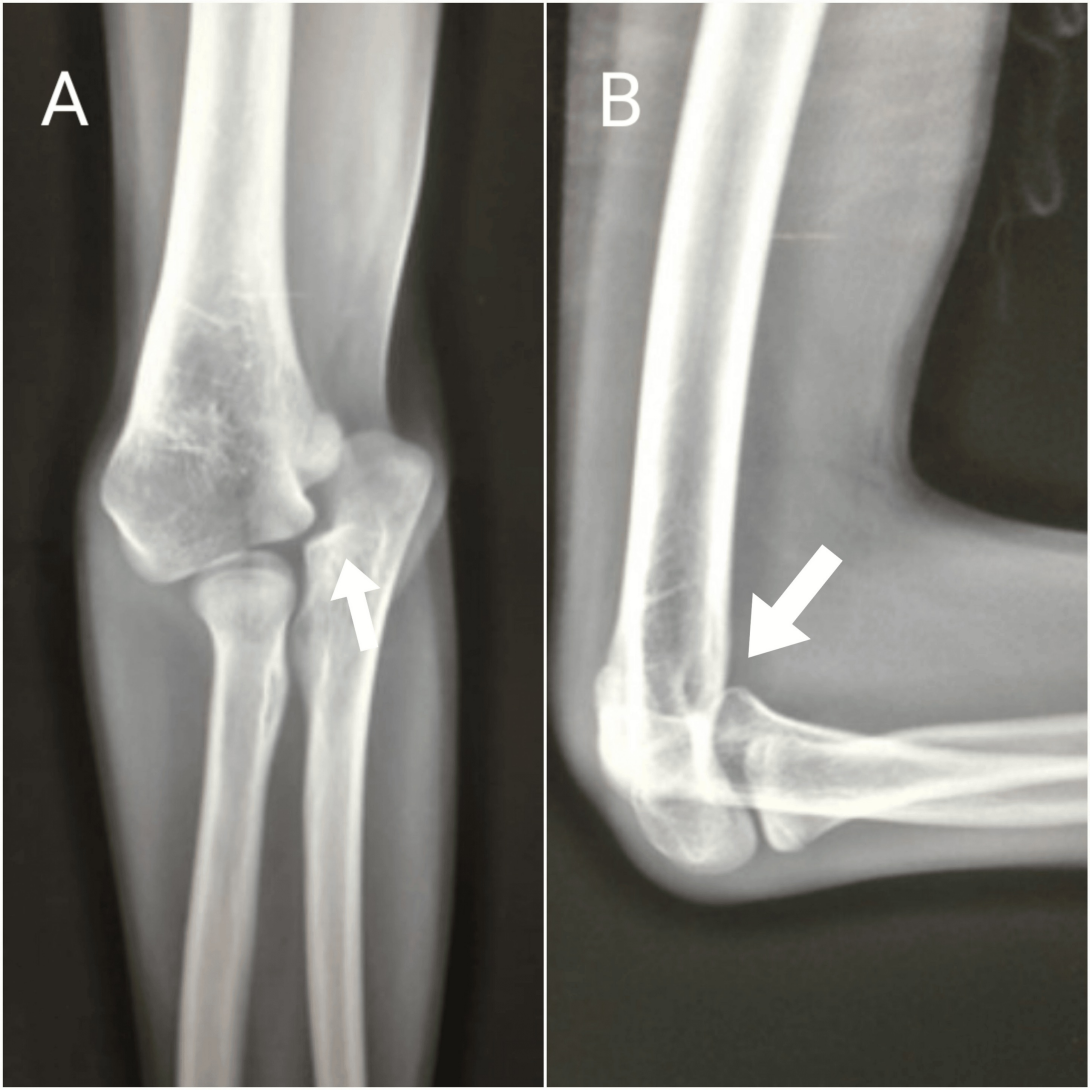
Right elbow X-rays at the 11-year follow-up, showing a preserved radio-humeral allignment Note the medial dislocation of the proximal ulna (arrow). A: Anteroposterior view. B: Lateral view
